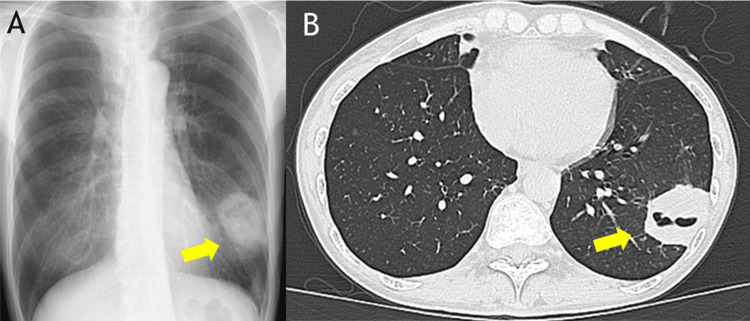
Imaging findings on admission (X year Y month) (A) Chest X-ray showing a newly appeared mass shadow in the left lower lung field (yellow arrow). (B) Chest CT scan showing a newly developed cavitary lesion with wall thickening in the left lower lobe (yellow arrow). CT: computed tomography
